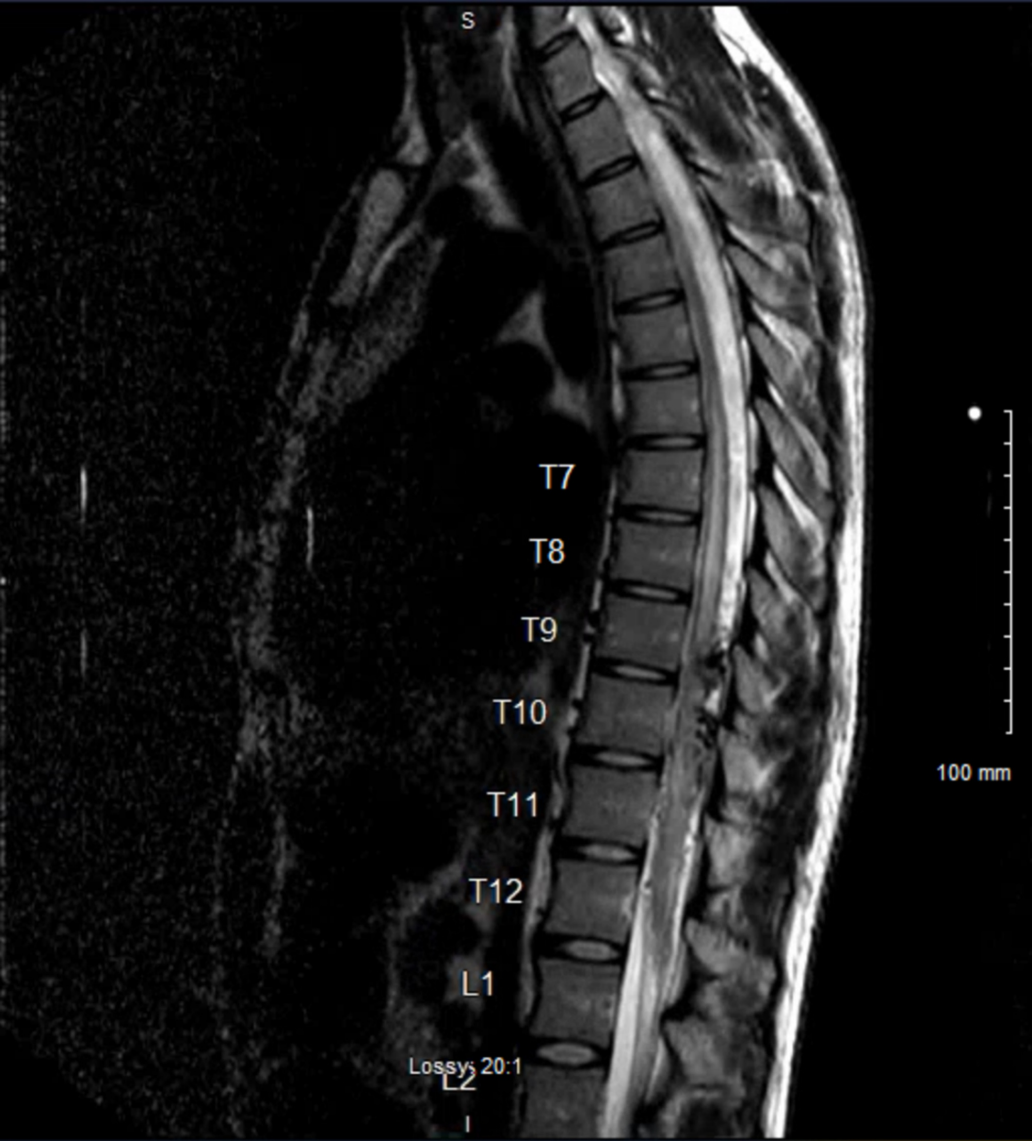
Preoperative MRI with and without contrast, T2 weighted.
